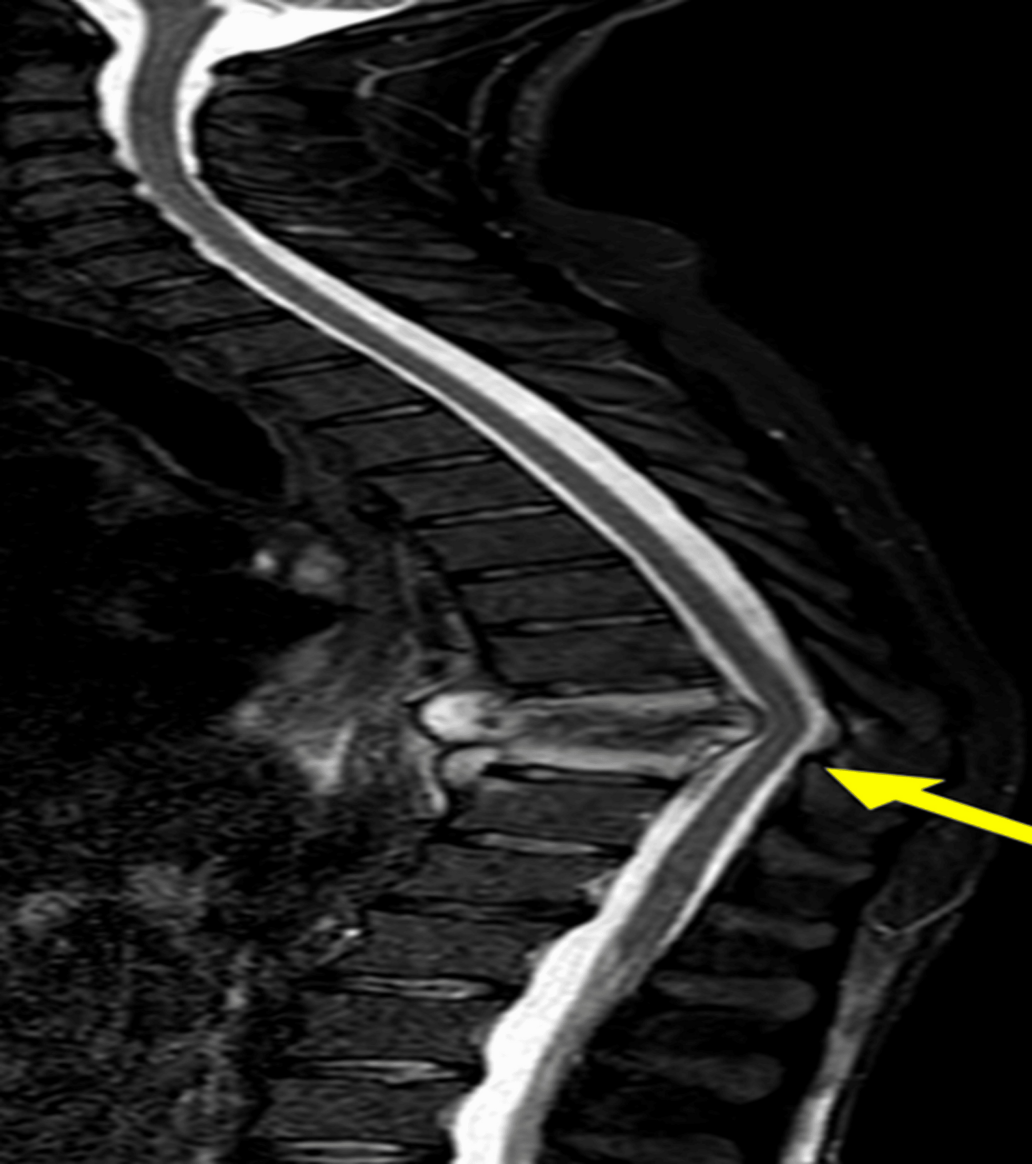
Spinal tuberculosis STIR: short tau inversion recovery; MRI: magnetic resonance imaging Sagittal STIR MRI sequence showing partial destruction of the T9 and T10 vertebral bodies, with edema and destruction of their endplates and the intervertebral disc due to T9-T10 spondylodiscitis (arrow) in a patient with disseminated tuberculosis and thoracolumbar pain. Diagnosis confirmed by MRI-guided vertebral biopsy showing caseating granulomas and culture-positive for *Mycobacterium tuberculosis*
